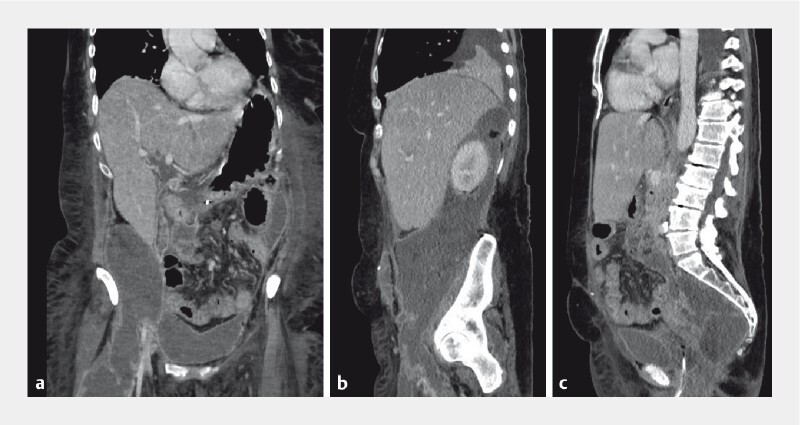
Contrast-enhanced computed tomography image of the abdomen 36 days after the onset of pancreatitis showing the distribution of necrosis in the:
